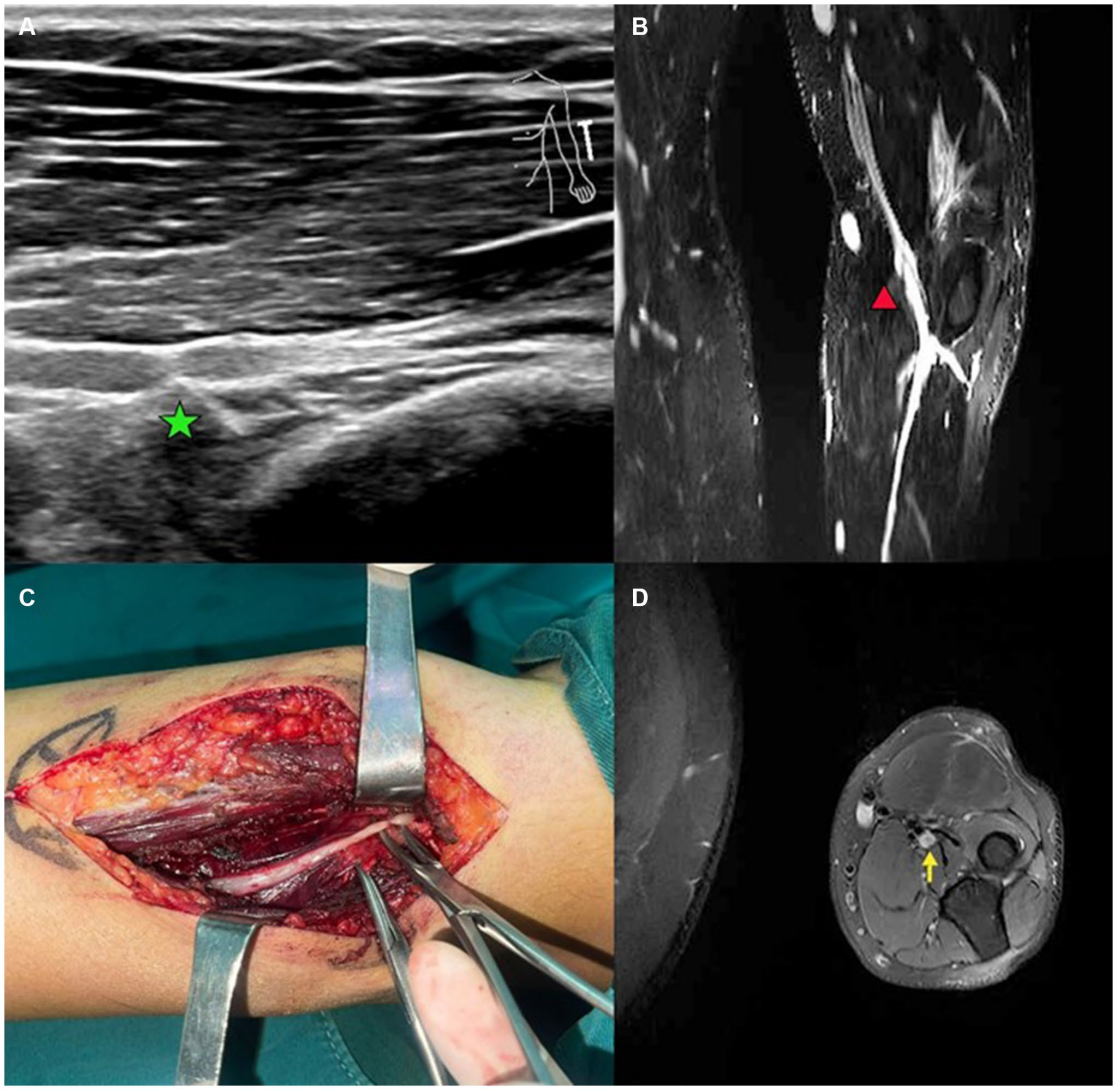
A case of patient with radial nerve entrapment. (A) US imaging of the radial nerve. Green star marks the proximal site of segmental entrapment. (B) Functional MRI of the radial nerve. Red triangle marks the site of radial nerve swelling. (C) Intraoperative image of deep branch of radial nerve. Segmental entrapment is observed. (D) MRI image of entrapped radial nerve. Yellow arrow makes the radial nerve swelling.
